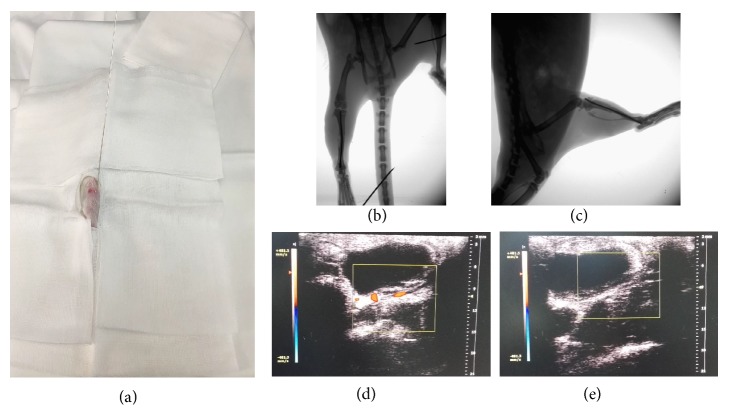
These images show the making process of fracture model and repetitive brief ischemia. (a) A sterilized 0.8 mm steel K-wire (Zimmer, USA) was used to enter the medullary cavity through the cortical bone by touching the leading edge of the tibial plateau when the keen was bent 90°. The wire stabbed directly the skin and was driven into medullary canal up to the distal of tibia. (b)-(c) X-ray examinations were performed to document the fracture reduction and the position of the implant ((b) anterior-posterior view; (c) lateral view). (d)-(e) Color doppler ultrasound (Vevo2100 imaging system, Canada) confirmed tourniquet pressure selected to block the blood ((d) tourniquet inflated; (e) tourniquet deflated).
